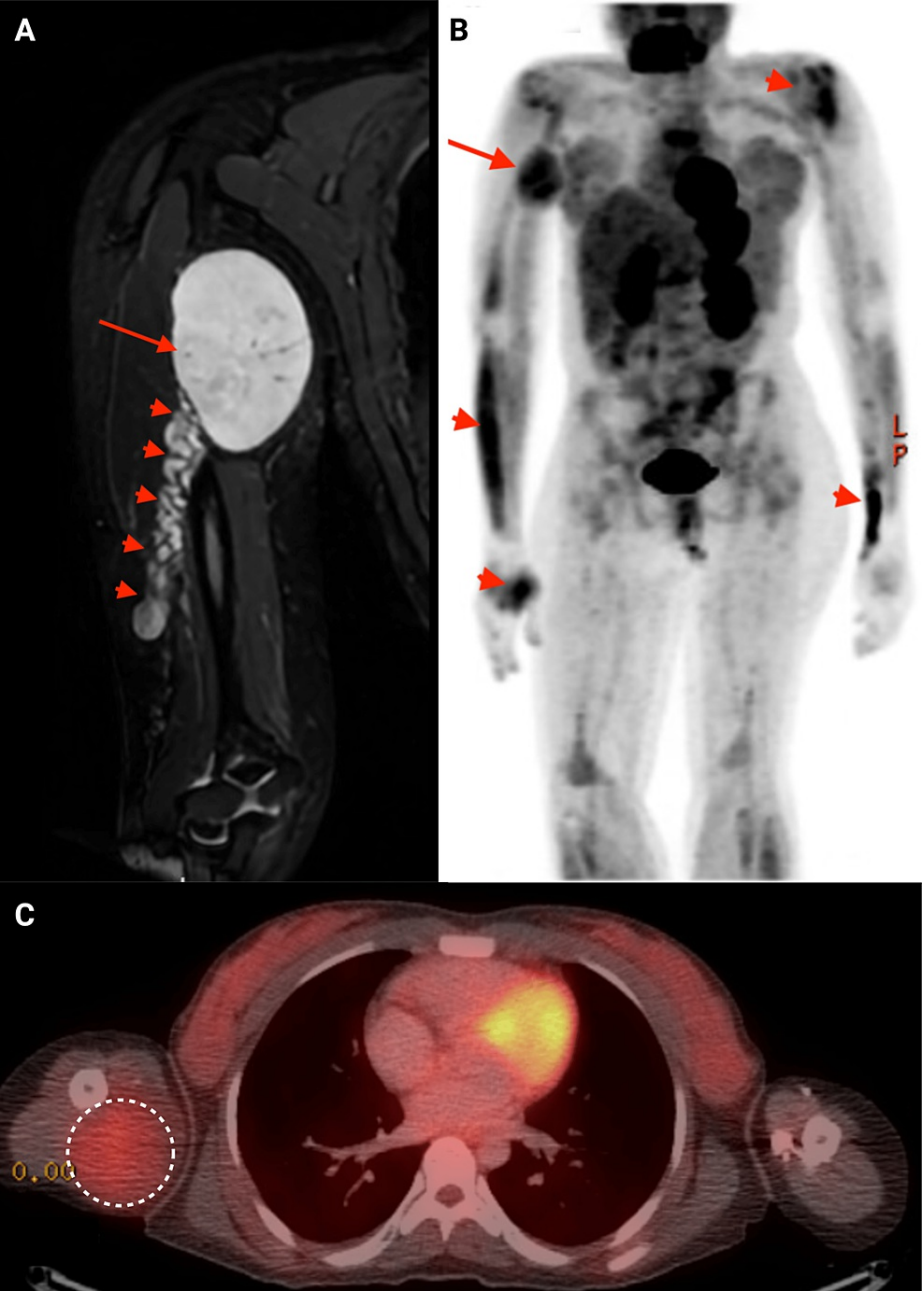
(A) Short tau inversion recovery (STIR) sequence magnetic resonance imaging (MRI) of the right upper extremity showing a large oval hyperintense mass (arrow) originating from the radial nerve, obstructing venous return due to the mass effect, with distal varicose veins (arrow heads). The mass shows increased uptake of 18-fluorodeoxyglucose (FDG) tracer in positron-emission tomography (PET) scan (B, arrow) and PET-CT (C, outlined). Several smaller masses with increased FDG uptake seen in upper extremities (B, arrow heads).
